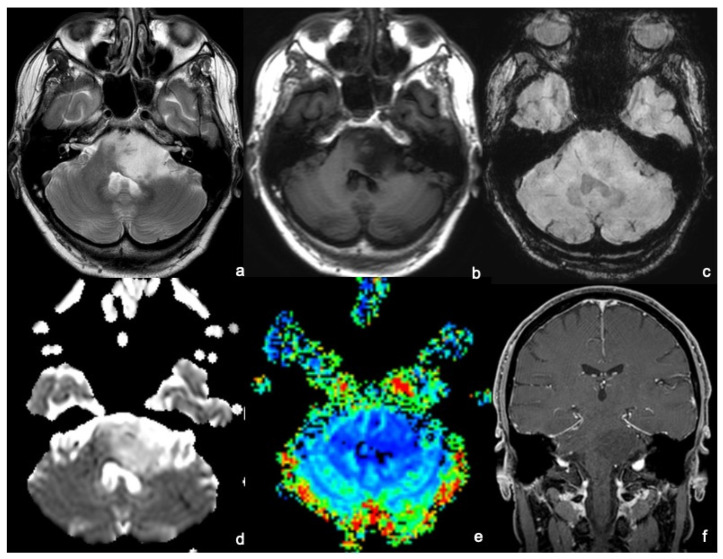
An MRI of a 45-year-old man presenting with a long-lasting headache and dizziness related to a diffuse intrinsic low-grade glioma. MRI shows a bulky lesion of the left pons extending: anteriorly, partially obliterating the prepontine cistern and contacting the patent basilar; posteriorly to invade the left–middle cerebellar peduncle and to compress the IV ventricle; and medially to the right hemipons. The lesion appears to be inhomogeneously hyperintense on T2WI (a) and hypointense on T1WI (b), without hemorrhagic foci on SWI (c), and without diffusion restriction foci on the ADC map (d). After gadolinium administration, the tumor does not enhance on T1WI (f), and there is no increased perfusion on the rCBV map (e). The anatomopathological analysis demonstrated that the lesion was a grade 2—2021 WHO classification of brain tumors.
